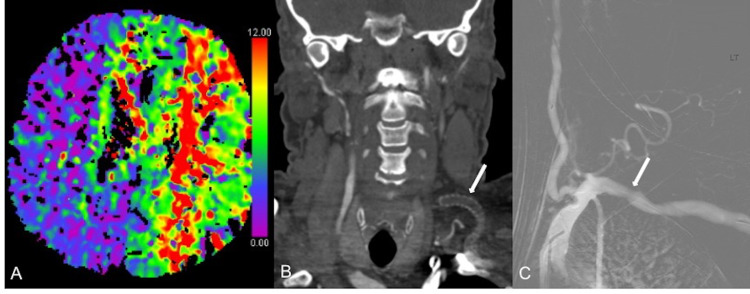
Stroke Imaging CT perfusion demonstrates a large area of ischemic penumbra involving the entire left anterior circulation and right anterior cerebral artery territory (A). CT angiogram of head and neck (coronal plane) shows complete occlusion of the left subclavian to ICA bypass graft (B, arrow). Angiographic image confirms complete occlusion of the bypass graft (C, arrow).
